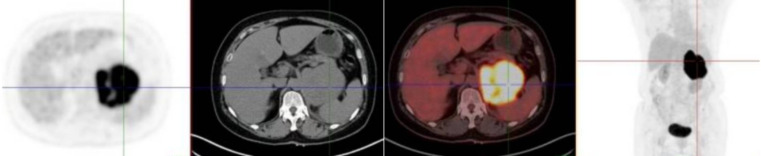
PET-CT image. Coronal PET-CT demonstrates significant FDG uptake in the left adrenal mass (SUVmax 14.3), with no evidence of distant metastasis.
